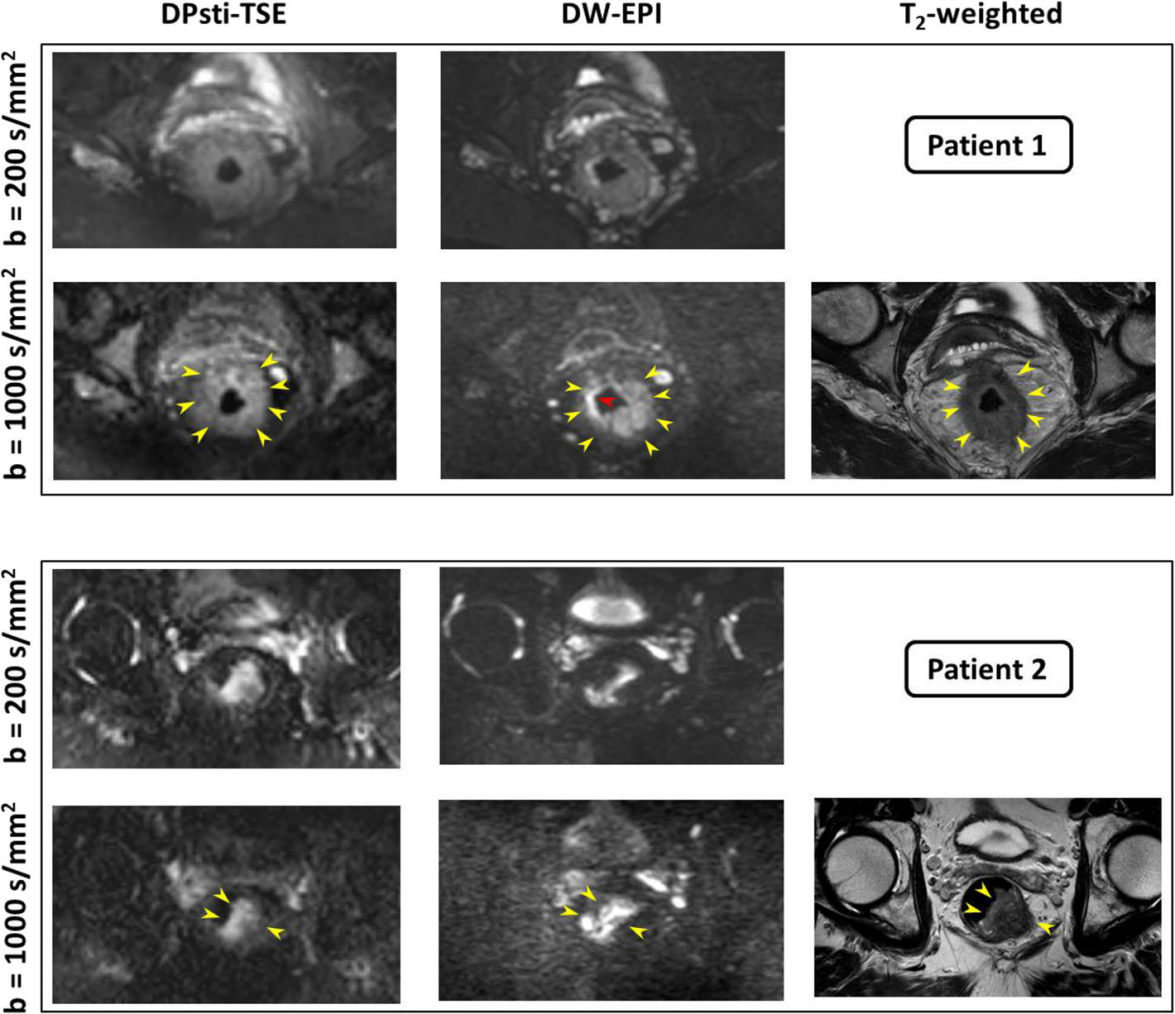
Representative images for two locally advanced rectal cancer patients. Tumours are indicated by yellow arrows, which are hyperintense on the b = 1000 s/mm^2^ images and hypointense on T2-weighted images. Intra-rectal gas led to minor signal pileup in DW-EPI images for patient 1 (red arrow) but more severe tumour distortion in DW-EPI images for patient 2. (*DPsti-TSE* diffusion-prepared stimulated echo turbo spin-echo, *DW-EPI* diffusion-weighted echo-planar imaging)
